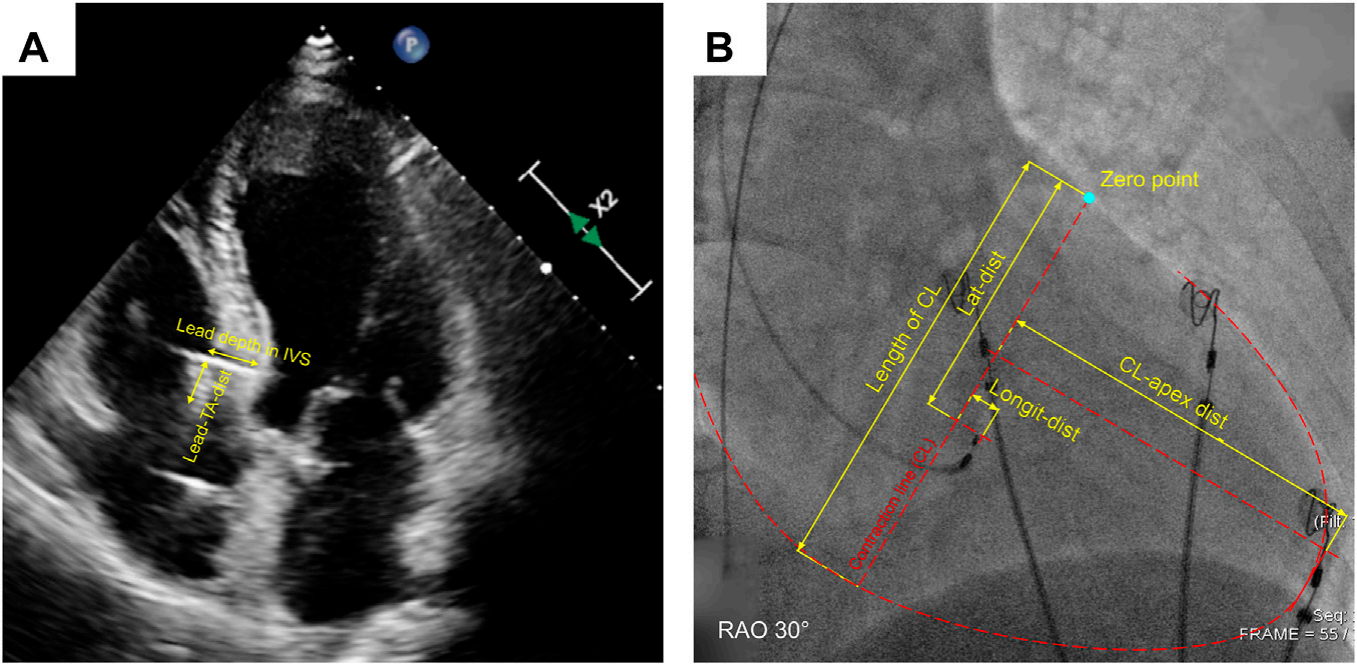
Measurement of echocardiographic (A) and fluoroscopic distance parameters (B). IVS, interventricular septum; Lead-TA-dist, distance from the lead-implanted site on the right surface of IVS to the septal leaflet of tricuspid annulus; CL, contraction line; CL-apex-dist, distance from CL to apex; Longit-dist, longitudinal distance; Lat-dist, lateral distance.
